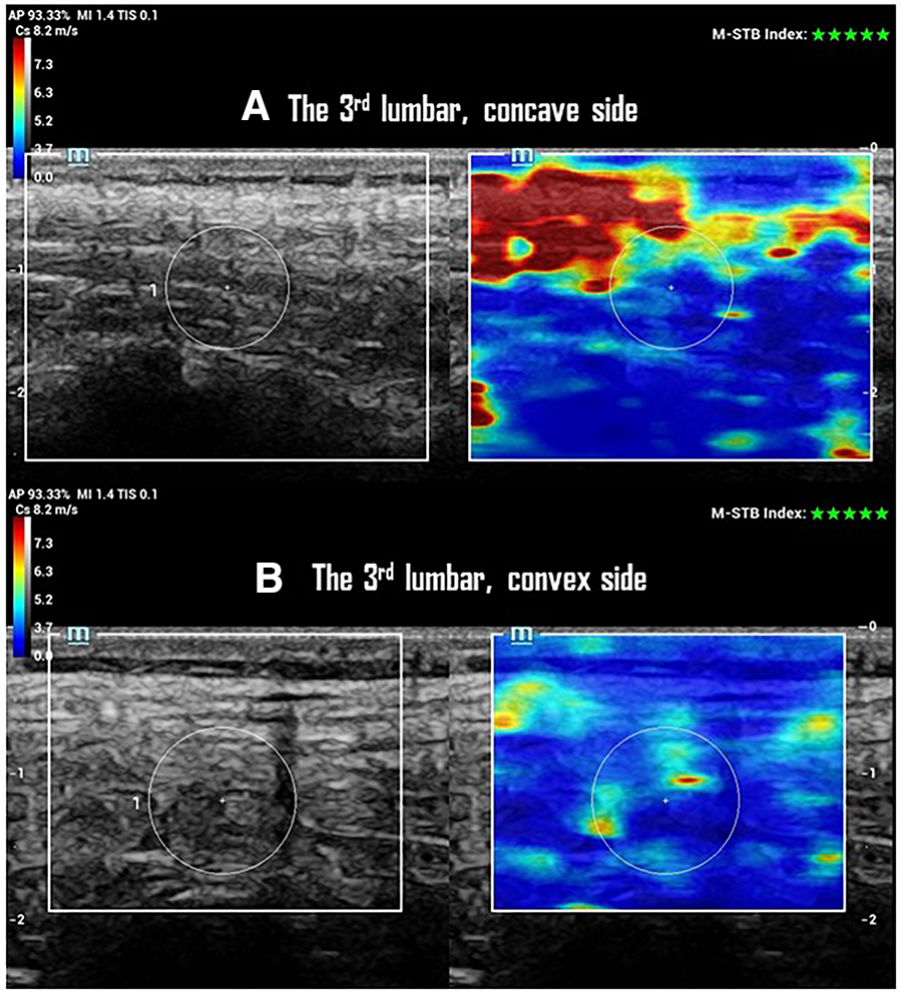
SWS and CTS of paraspinal multifidus at the 3rd lumbar vertebrae of one participant with a 25° left lumbar scoliosis in the progression group. (A) Ultrasonographic imaging shows a 10 mm CTS with 4.4 ± 1.4 m/s of SWS in multifidus on the concave side of the curve. (B) Ultrasonographic imaging shows a 10 mm of CTS with 3.9 ± 1.0 m/s of SWS in multifidus on the convex side of the curve.
